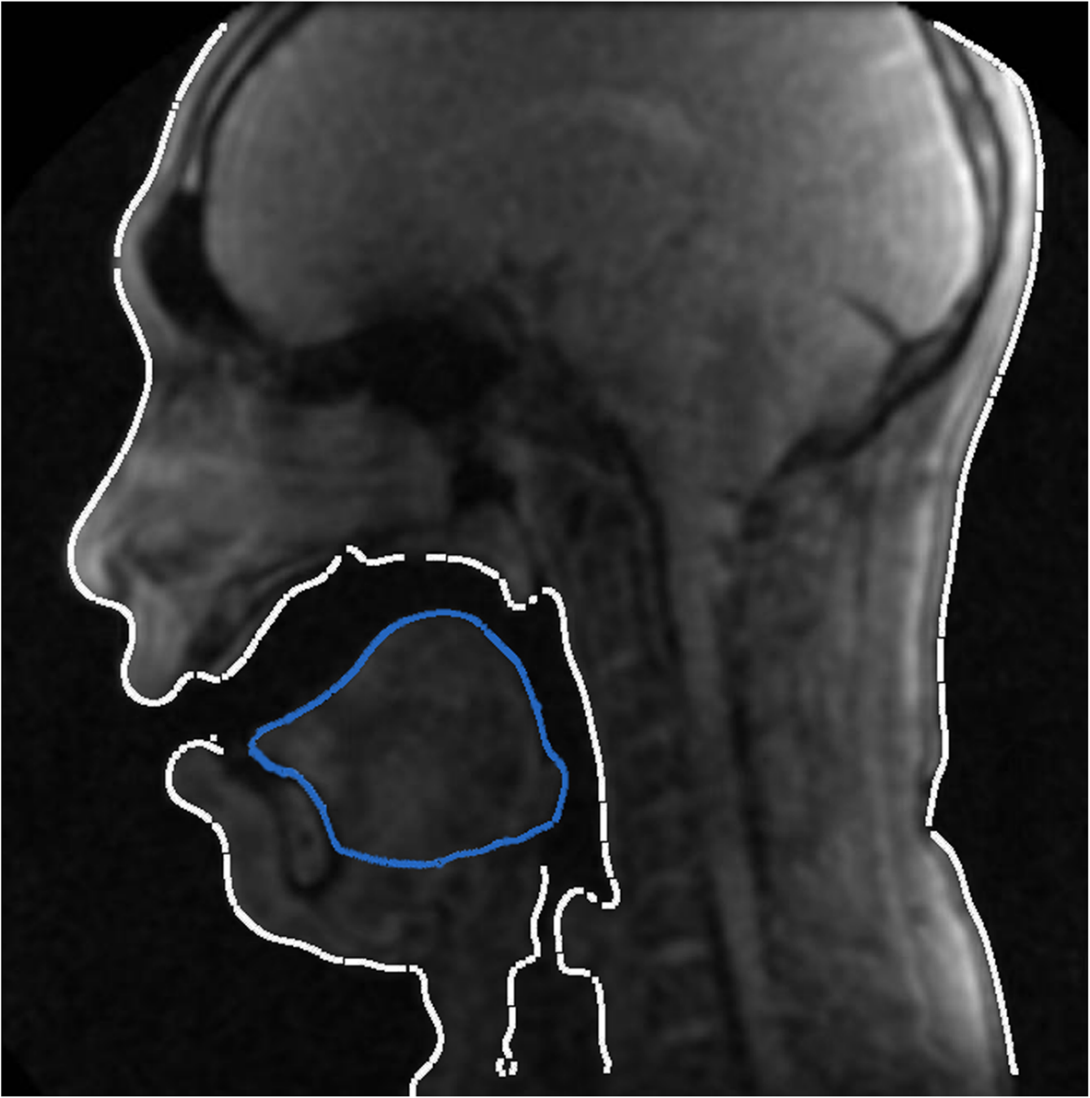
A sample of MRI frame of male subject. The contours provided by the proposed method. The blue contours have been provided from the active contour while the white contours obtained from preprocessing.
